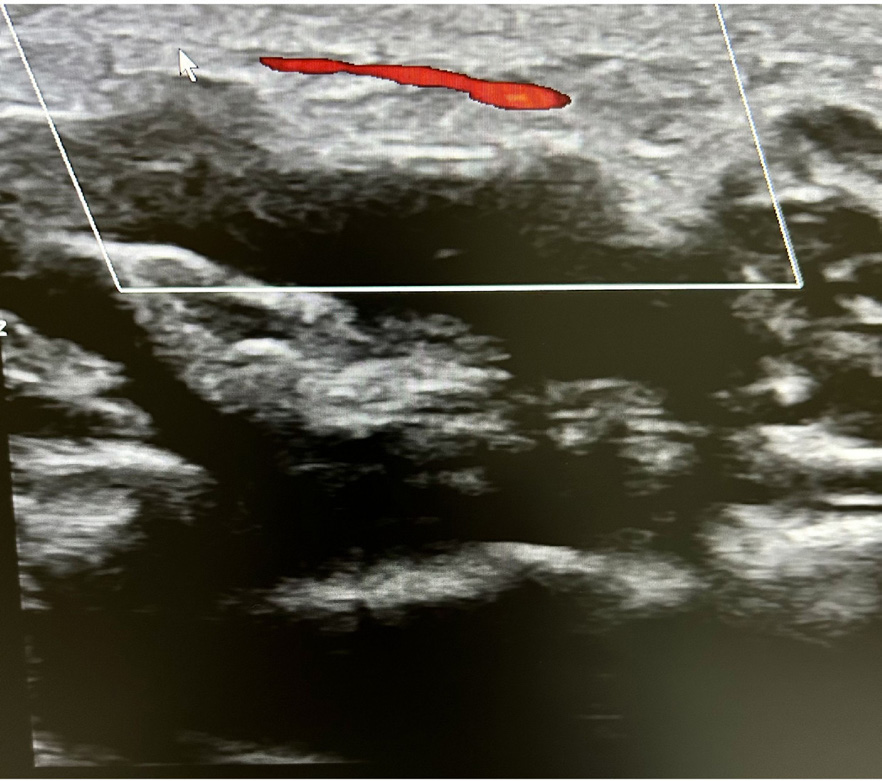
Superficial palmar arch patent on color Doppler ultrasonography.
